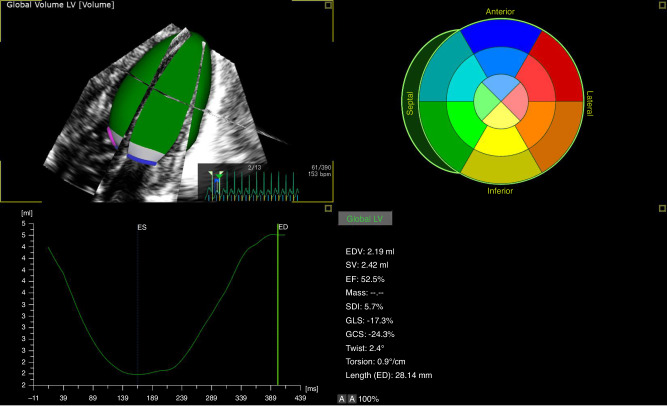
Offline analysis of three-dimensional echocardiographic left ventricular (LV) volume curve and function calculations. *ED* end-diastole, *ES* end-systole, *EDV* end-diastolic volume, *ESV* end-systolic volume, *SV* stroke volume, *EF* ejection fraction, *SDI* systolic dyssynchrony index, *GLS* global longitudinal strain, *GCS* global circumferential strain.
